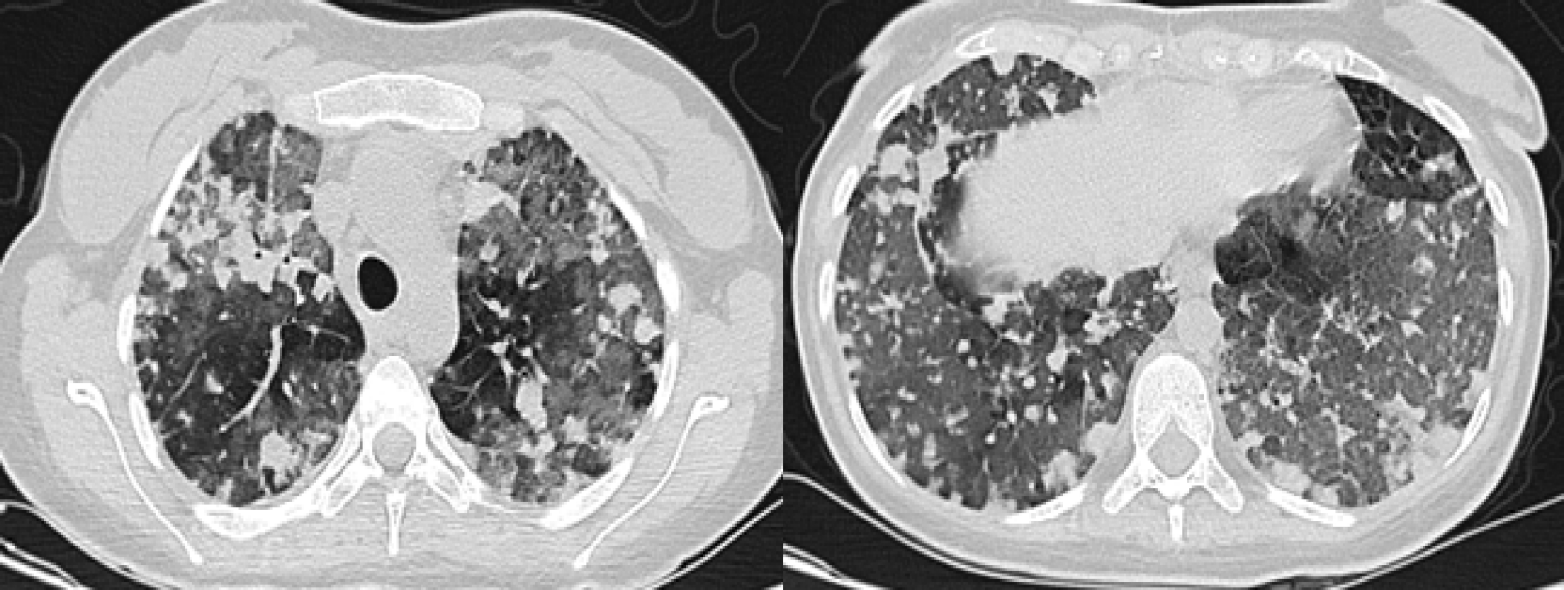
A computed tomography chest scan revealed numerous nodular opacities of varying sizes and extensive alveolar haziness, consistent with diffuse alveolar haemorrhage.
